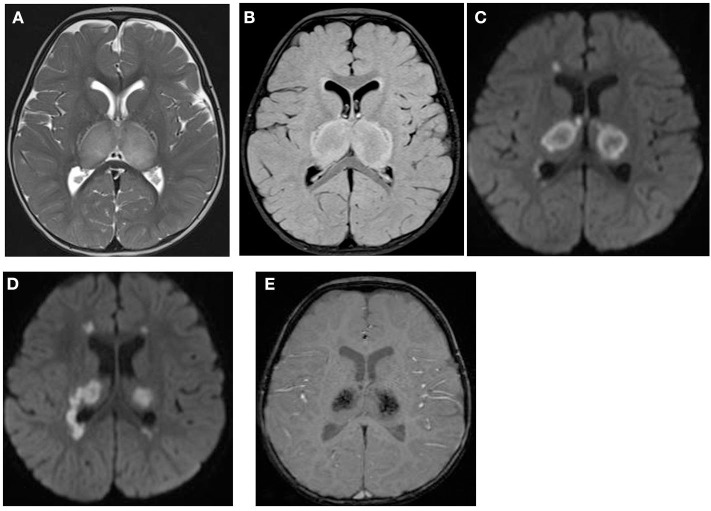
Brain MRI. Axial T2 (A), FLAIR (B), diffusion (C,D), and susceptibility (E) weighted images. There are swelling and abnormal high signal intensity of both thalami (A,B) with diffusion restriction (C,D) and dark signal intensity with blooming (E).
